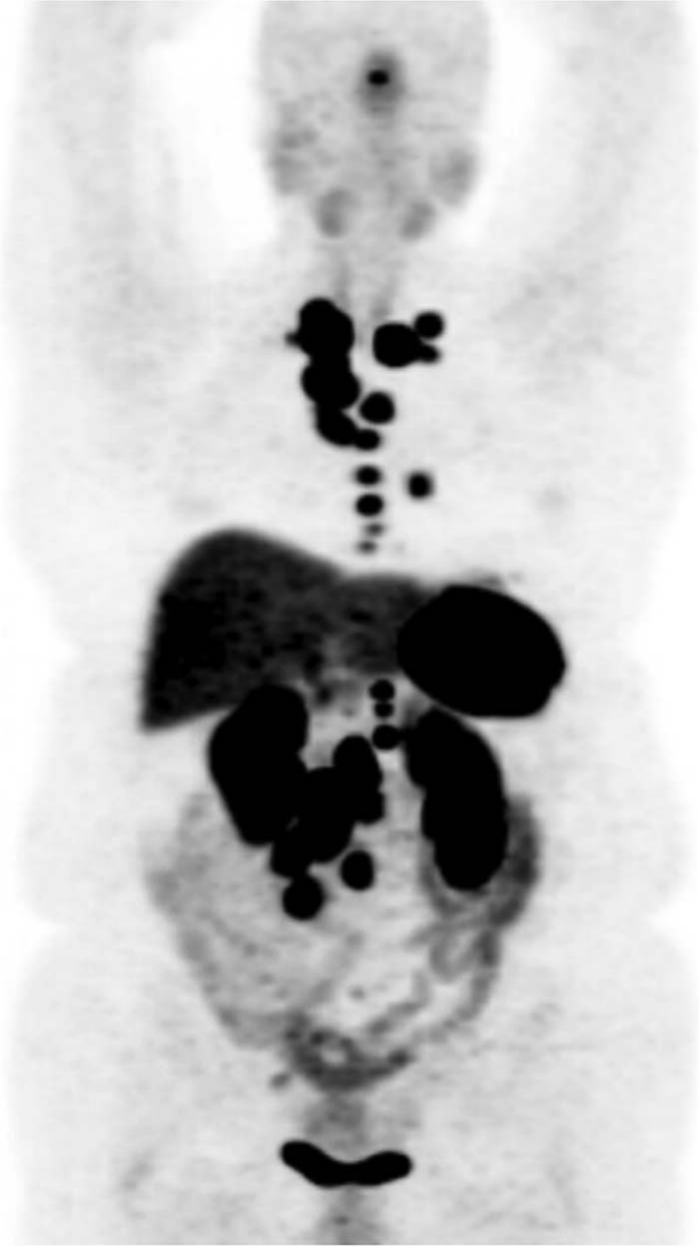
DOTATATE positron emission tomography-computed tomography scan revealing extent of metastatic disease.
